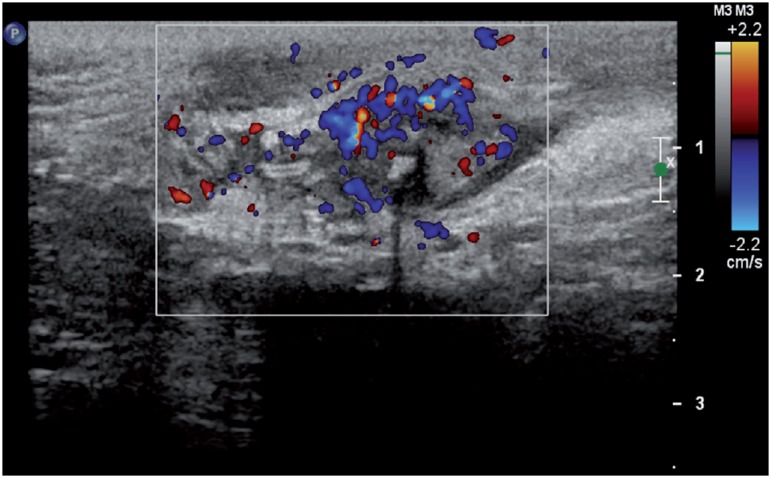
Color Doppler sonography of the granuloma shows the hypervascularity of the lesion.
